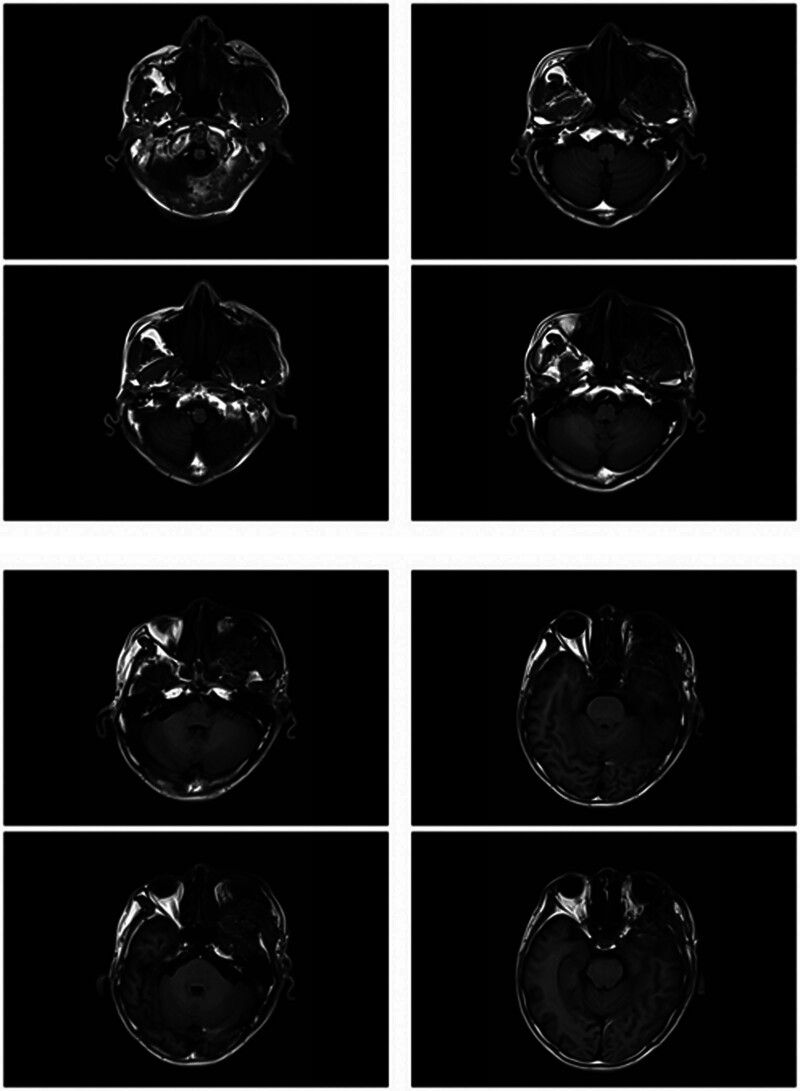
Patient postoperative MRI cross-sectional T1 phase. MRI = magnetic resonance imaging.
